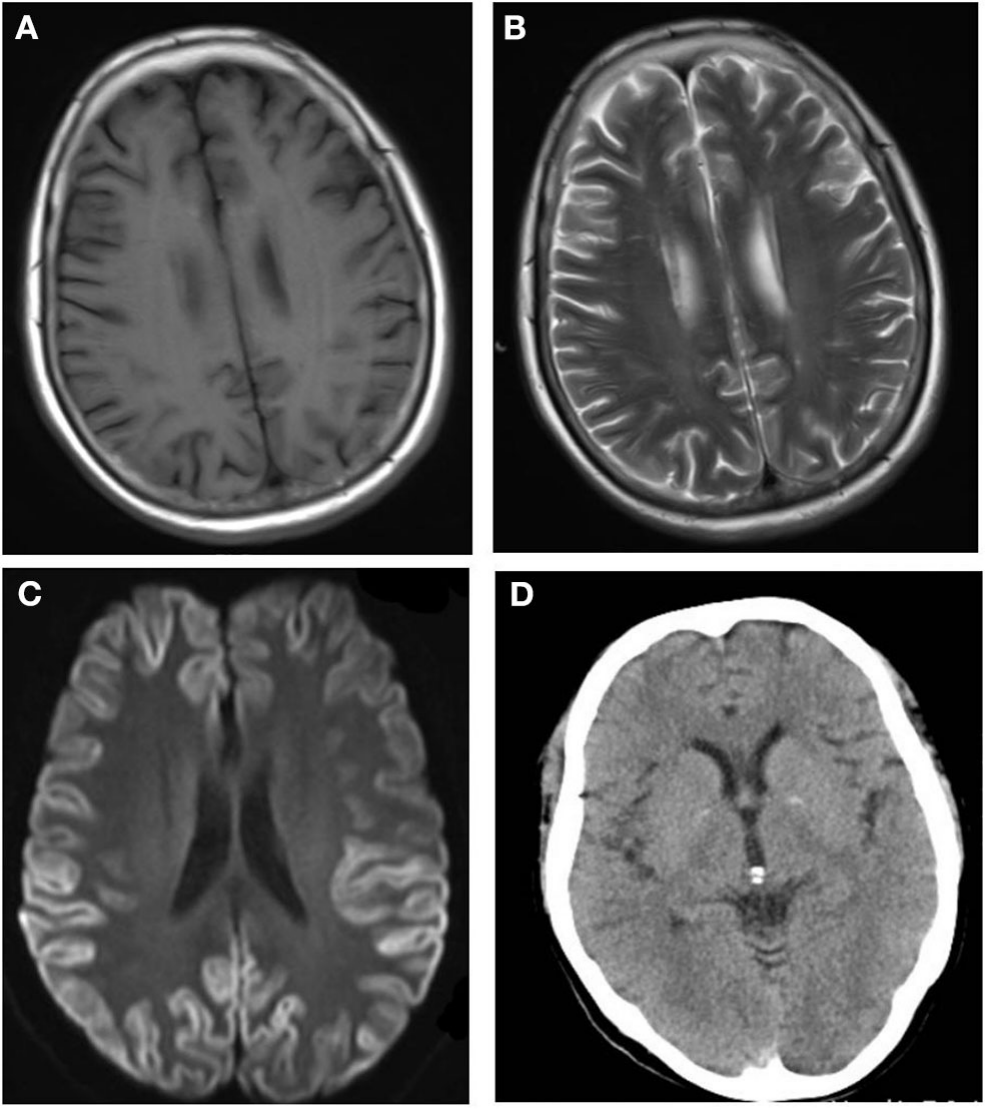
No abnormalities are detectable on the T1-weighted (A) and T2-weighted (B) magnetic resonance imaging (MRI) sequences. The diffusion-weighted imaging (DWI; C) shows the typical features of “cortical ribboning” in the cortex and subcortex of bilateral frontal, temporal, and occipital lobes on DWI (C). On the apparent diffusion coefficient map (ABC; D), there is low signal intensity in the cortex and subcortex of the bilateral frontal, temporal, and occipital lobes.
